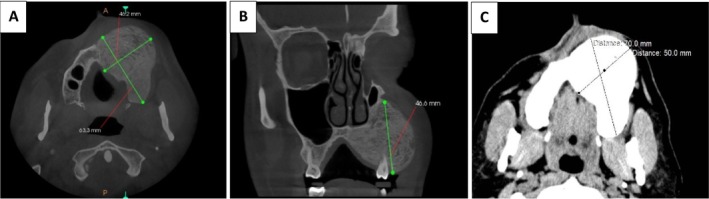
CT of the lesion after COVID‐19 infection in two intervals. (A, B) One year after the infection. (C) Two years after the infection.
